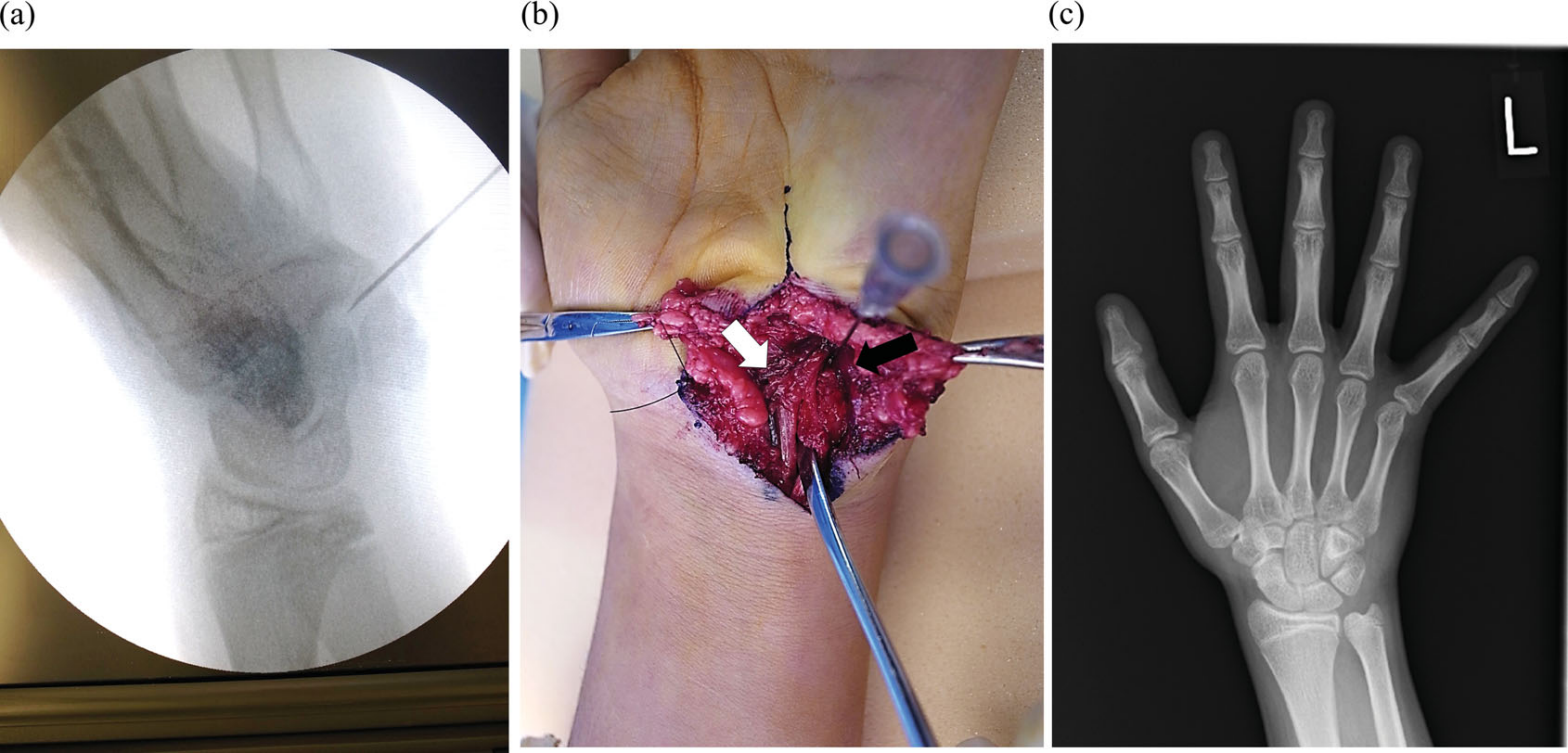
(a) X-ray imaging during the surgery; the needle was inserted between pisiform and hamate (b) Interoperative view; ulnar nerve (white arrow) is retracted radial to the coalition (black arrow). The nerve runs over the hook of the hamate. (c) Plane X-ray two years after the surgery.
